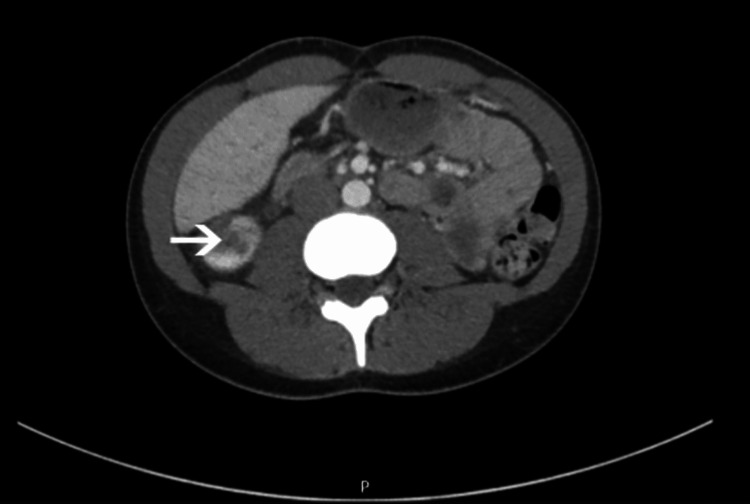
Contrast-enhanced abdominal computed tomography showing a hypodense region in the central right kidney (white arrow).
